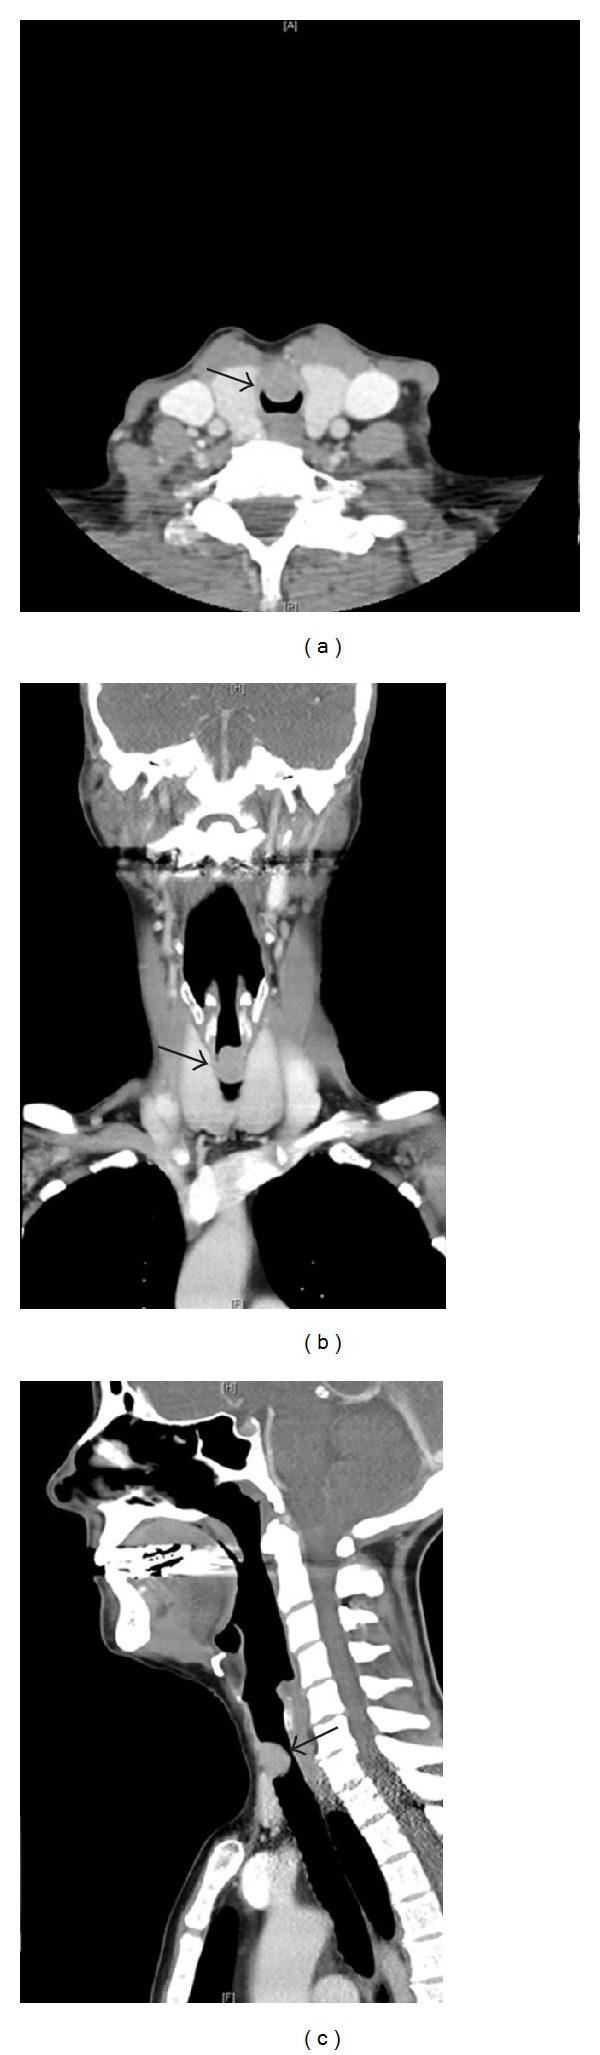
(a) CT neck with intravenous contrast in axial plane, (b) coronal plane, and (c) sagittal plane demonstrating a 1.3 × 1.4 × 1.9 cm round soft tissue mass (arrow) extending posteriorly from the anterior wall of the upper trachea at the level of C6, approximately 2 cm inferior to the true vocal cords associated with significant (>80%) airway obstruction.
